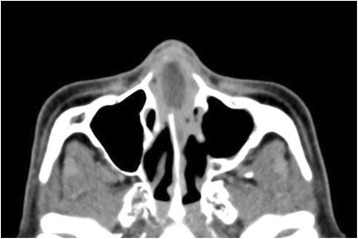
Axial view of contrast-enhanced computed tomography (CT). A swollen, ring-enhancing lesion of the anterior nasal septum was observed
